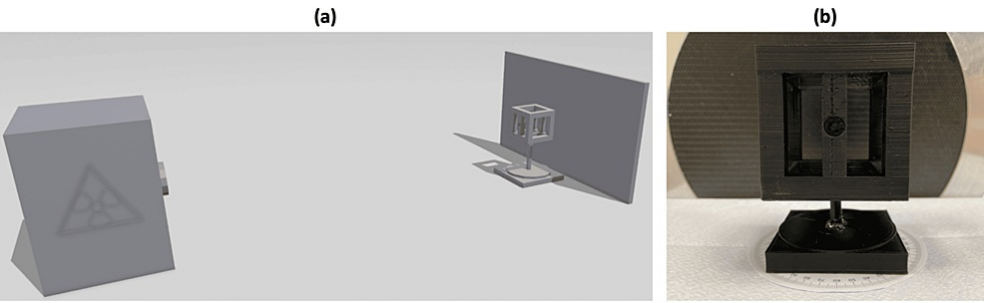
(a) A scheme showing the experimental setup with the 3D-printed prototype on a rotating platform 65 cm away from the radiation source (C-arm, X-ray). (b) 3D-printed prototype placed in front of the C-arm.
